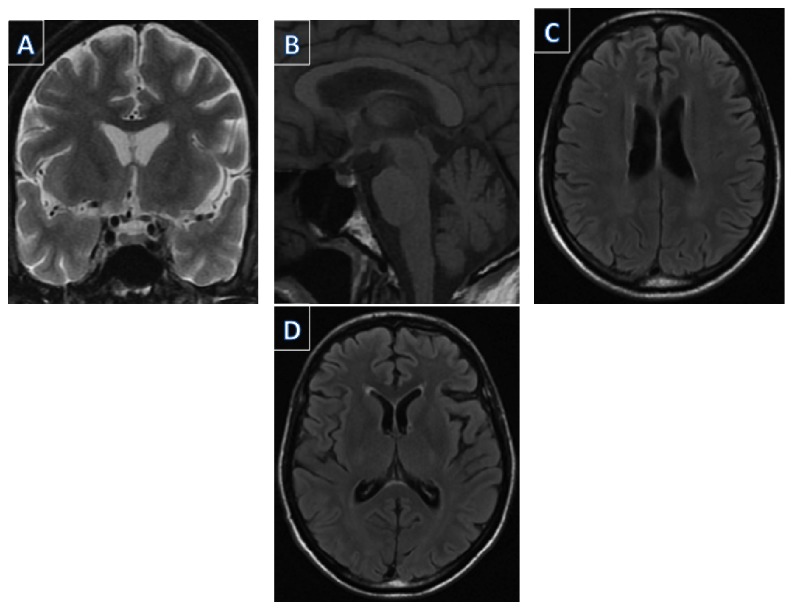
Magnetic resonance imaging of the brain was performed without significant alterations.
